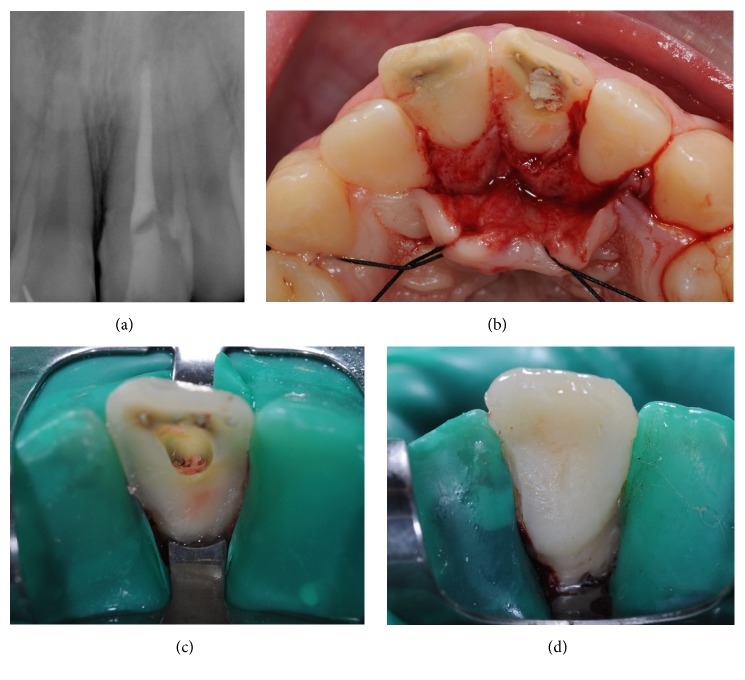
(a) Final periapical radiograph after pulpectomy. (b) Clinical aspect during the exploratory surgery showing the extension of the complicated crow-root fracture, invading the biological width in the palatal aspect. (c) After gingival flap and osteotomy, a rubber dam was placed. (d) Final aspect after fragment reattachment and polishing.
